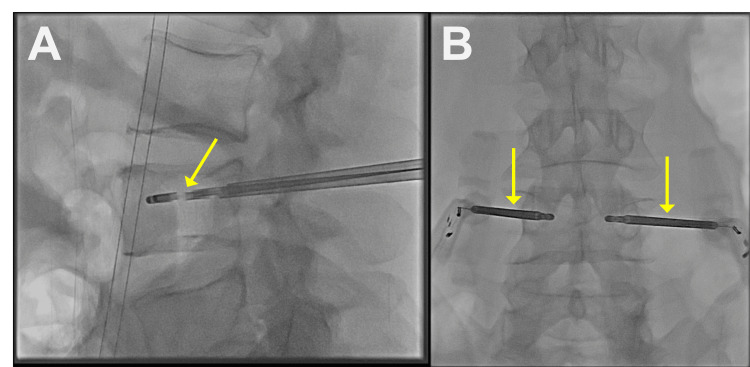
Computed tomography fluoroscopic imaging showing radiofrequency ablation probes inserted into the L4 vertebral body (yellow arrows)
